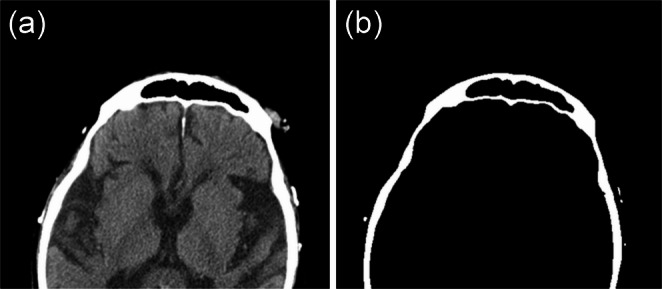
(a) A representative axial CT image showing the frontal sinus; (b) an image of (a) in which CT values less than 200 HU are displayed in black and those 200 HU and over in white
